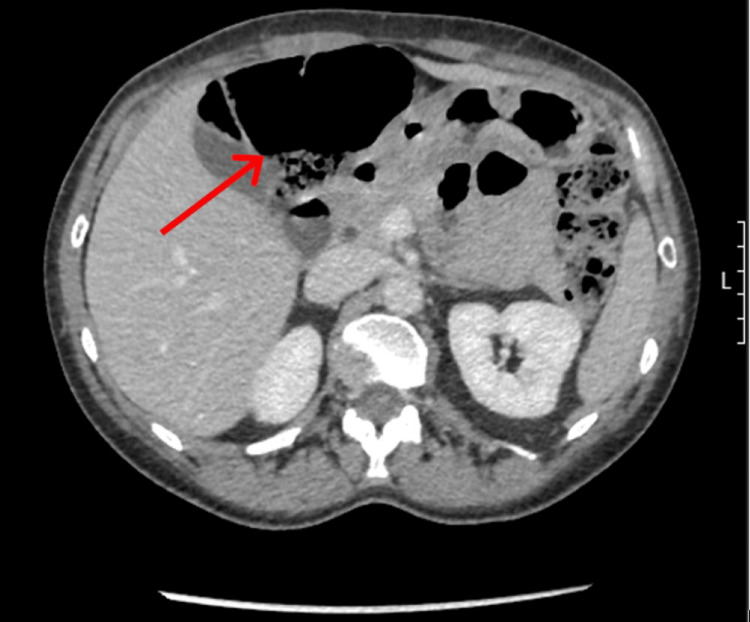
Transverse CT image of massively dilated bowel (solid arrow).
